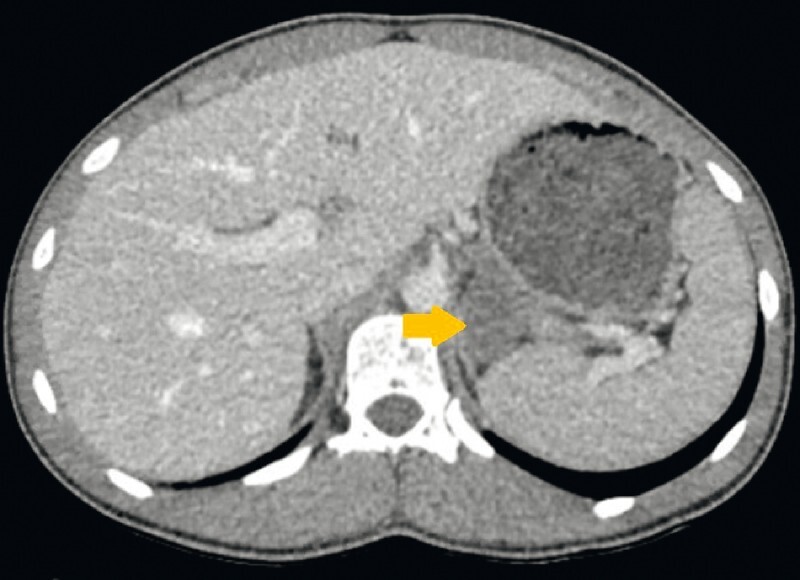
Abdominal computed tomography scan 4 months after the infectious episode, showing a decrease in size of the retro-gastric cystic lymphangioma (yellow arrow).
